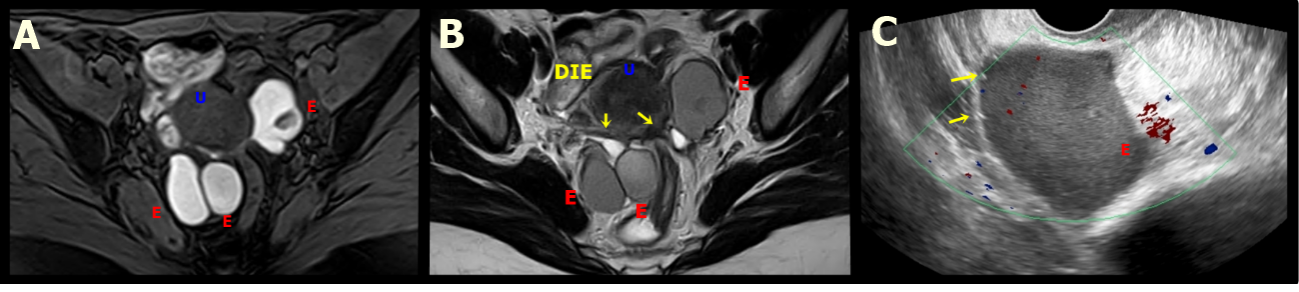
A. Axial T1W MR image with fat saturation shows bilateral ovarian endometriomas with marked and uniform hyperintensity with a “light bulb bright” appearance, demonstrating hematic content. U: Uterus. B. Axial T2W MR image shows bilateral ovarian endometriomas (E) with decreased signal on T2W (T2 shading) that reflects the varying chronicity of blood products from repeated haemorrhage. Yellow arrows show a deep endometriosis plaque in the retrocervical space, with extension to the left ovarian fossa and anterior rectal wall. U: UterusC. Transvaginal ultrasound showing a unilocular cyst with regular walls and homogeneous ground glass echogenicity, compatible with endometrioma (E) with small hyperechogenic focus in the wall (yellow arrow).
